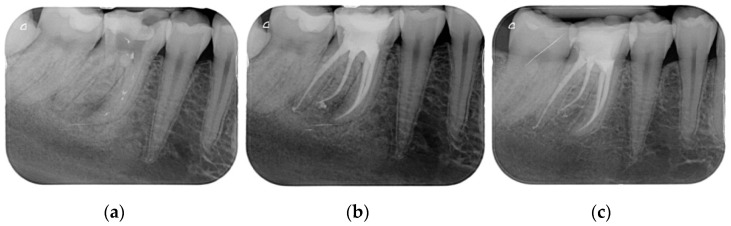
(a) A first lower molar with periapical lesion and in need to be retreated. (b) The molar immediately after being endodontically treated. Four canals (one in a radix entomolaris) were detected and treated. (c) The molar after 4 years. The good health of the periapical areas can be noted. The crown was restored with an adhesive esthetic onlay.
